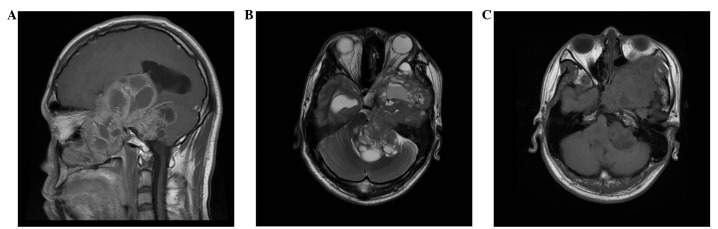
Case 3. (A) The T1-weighted soft-tissue mass was wide and large at the base of the anterior cranial fossa, and the surrounding tissues were extensively destroyed. (B) T2-weighted signal imaging occurred in the mass. (C) The solid components of the tumor were significantly enhanced.
